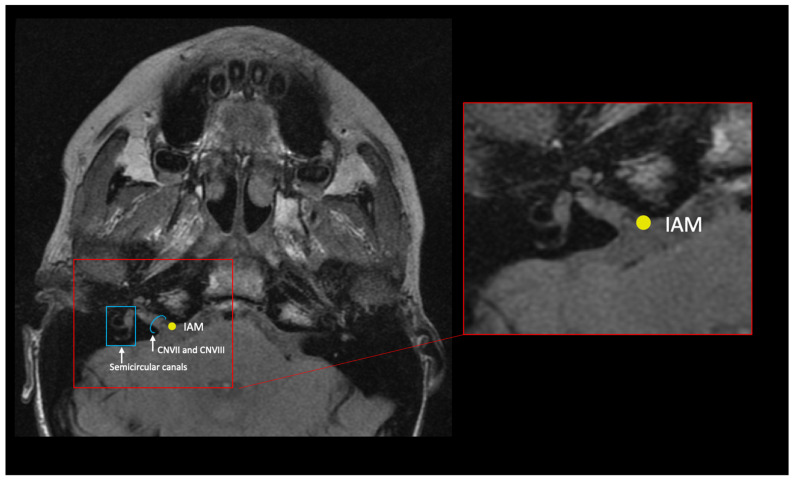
Oblique axial slice from an MRI scan depicting the internal acoustic meatus. CNVII: facial nerve (cranial nerve 7); CNVIII: vestibulocochlear nerve (cranial nerve 8); semicircular canals; IAM: internal acoustic meatus.
